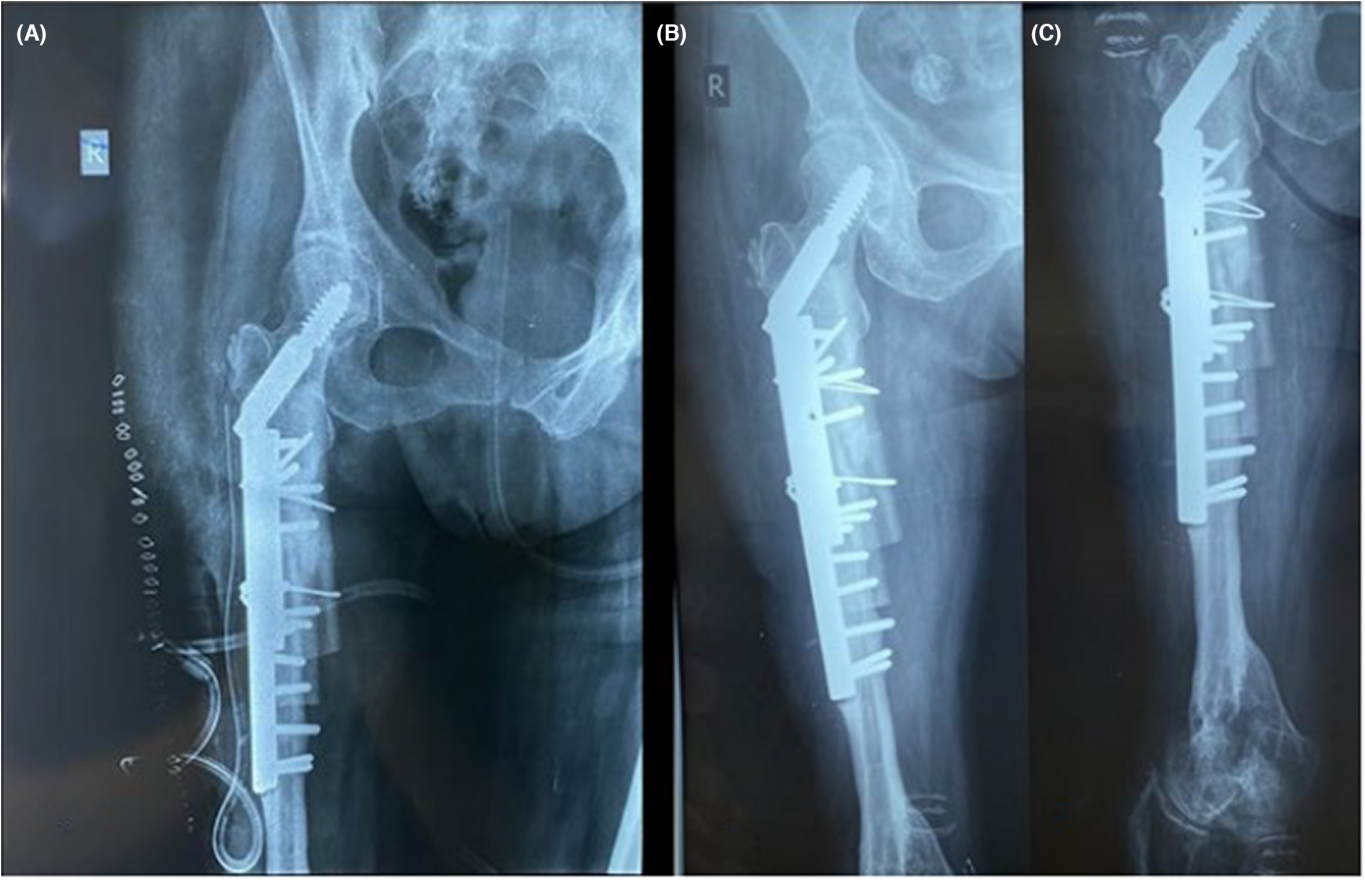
Postoperative radiographic imaging. (A) 1‐month post‐op, (B) 3‐month post‐op, and (C) 6‐month post‐op.
